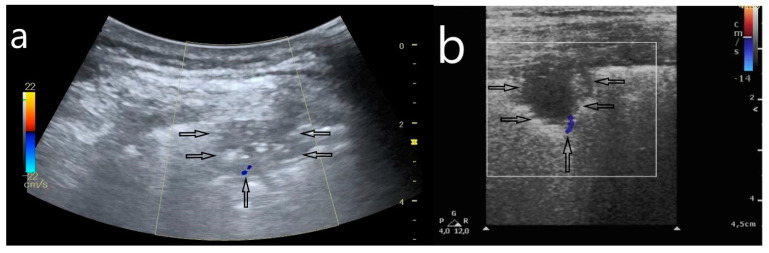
Subpleural consolidation due to pulmonary embolism secondary to COVID-19. (a,b) Wedge-shaped subpleural consolidation (horizontal arrows), with a ‘vascular sign’—flow amputation visible in Color Doppler (vertical arrow).
